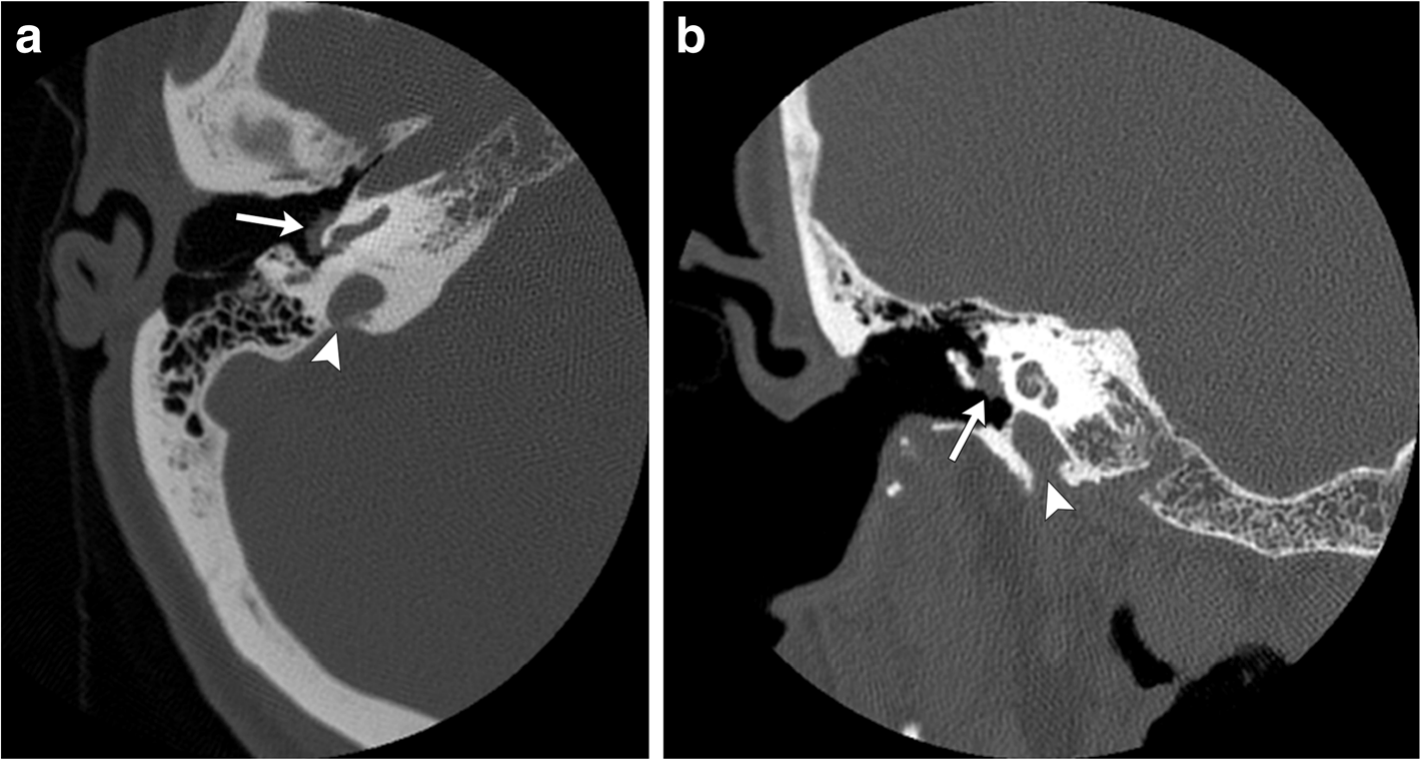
Axial (a) and coronal (b) CT images through the right temporal bone demonstrate a smooth, well-defined smooth soft tissue mass arising from the lateral aspect of the cochlear promontory, expanding outward into the middle ear (white arrows). Note the intact jugular bulb (white arrowhead)
